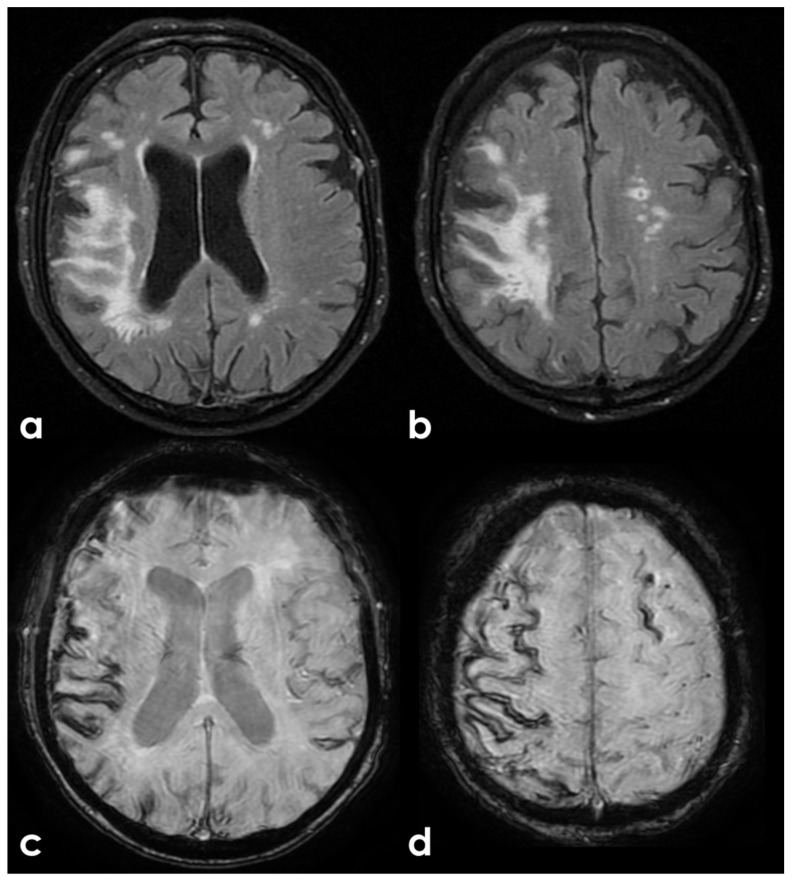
Control MRI, showing an increasing in white matter hyperintensity ion the right hemisphere (axial FLAIR, panels (a,b)) and an increased burden of cortical superficial siderosis (SWI in panels (c,d)) in the left superior frontal sulcus.
